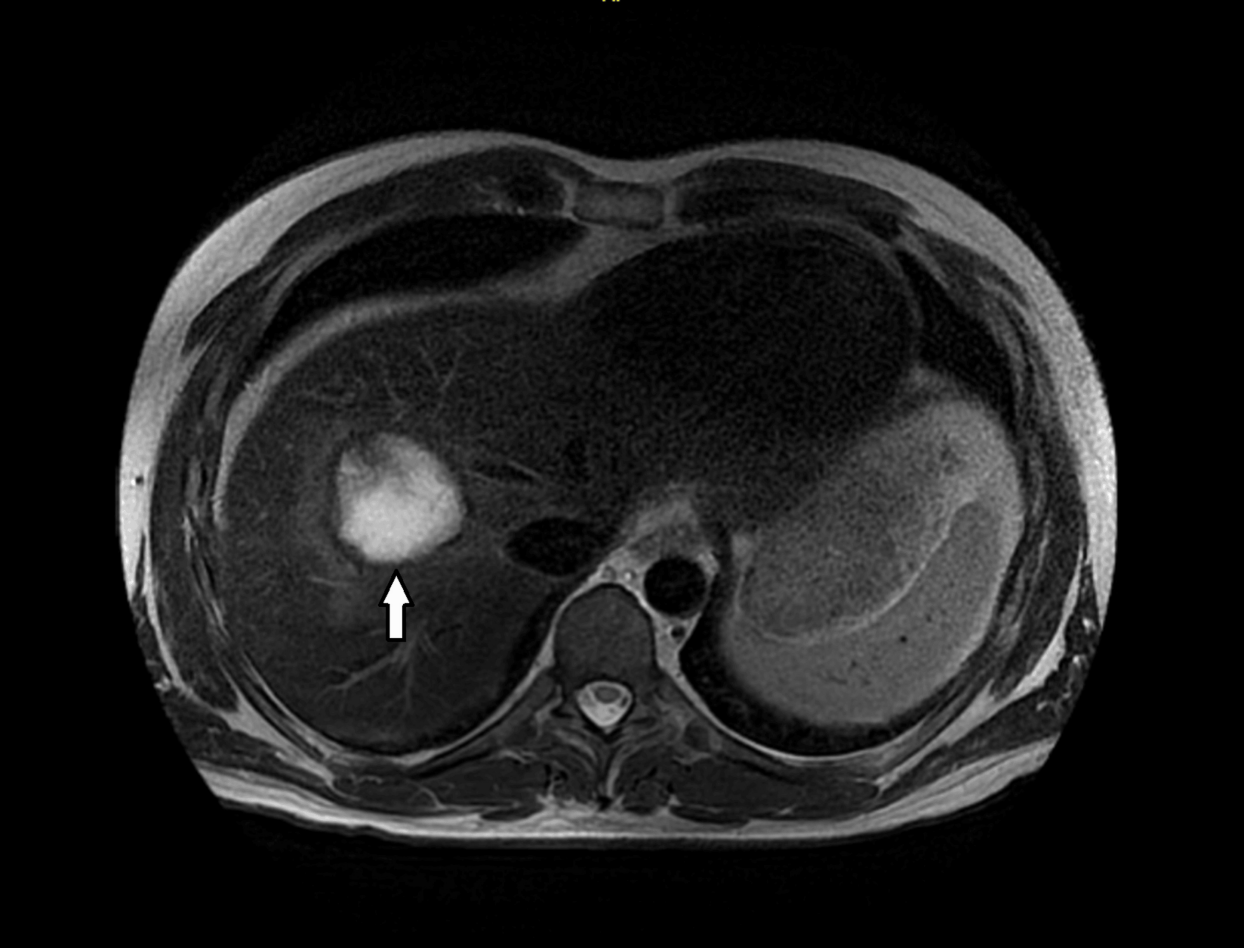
A magnetic resonance imaging scan with contrast of the abdomen. Post-ultrasound-guided drainage of the abscess showed reduction of the hepatic abscess measuring 3.2cm x 3.4cm (white arrow).
